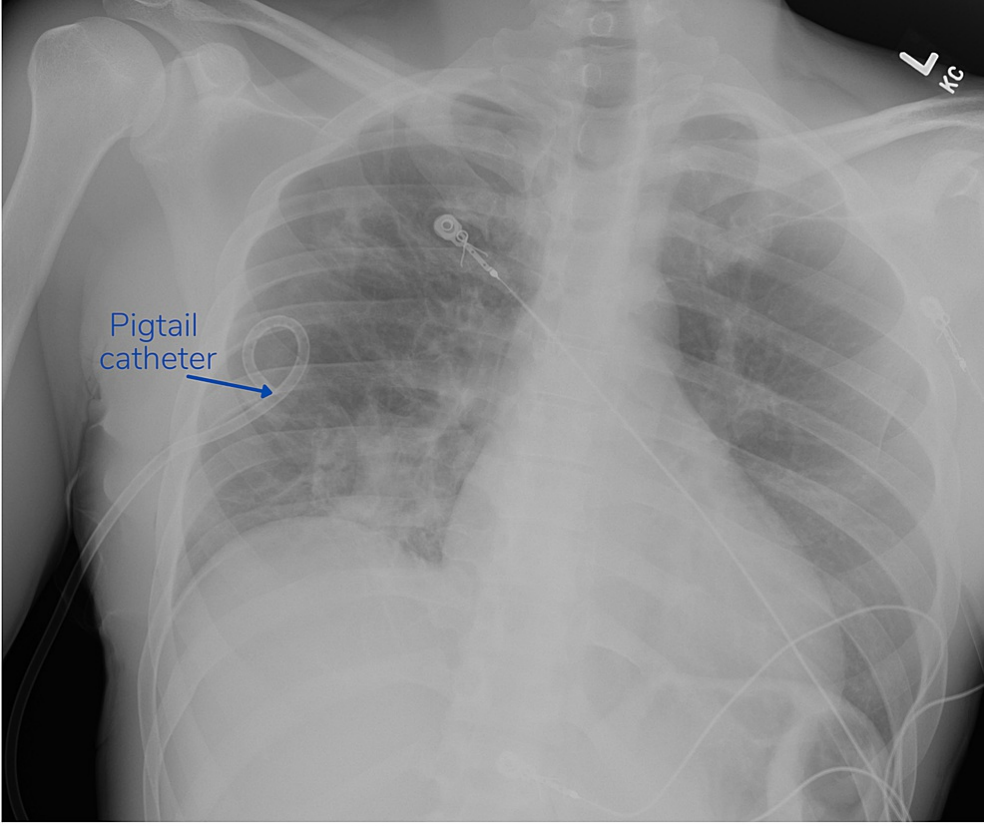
Chest radiograph taken two hours and twenty-five minutes after initial presentation demonstrating resolution of pneumothorax following pigtail catheter placement
